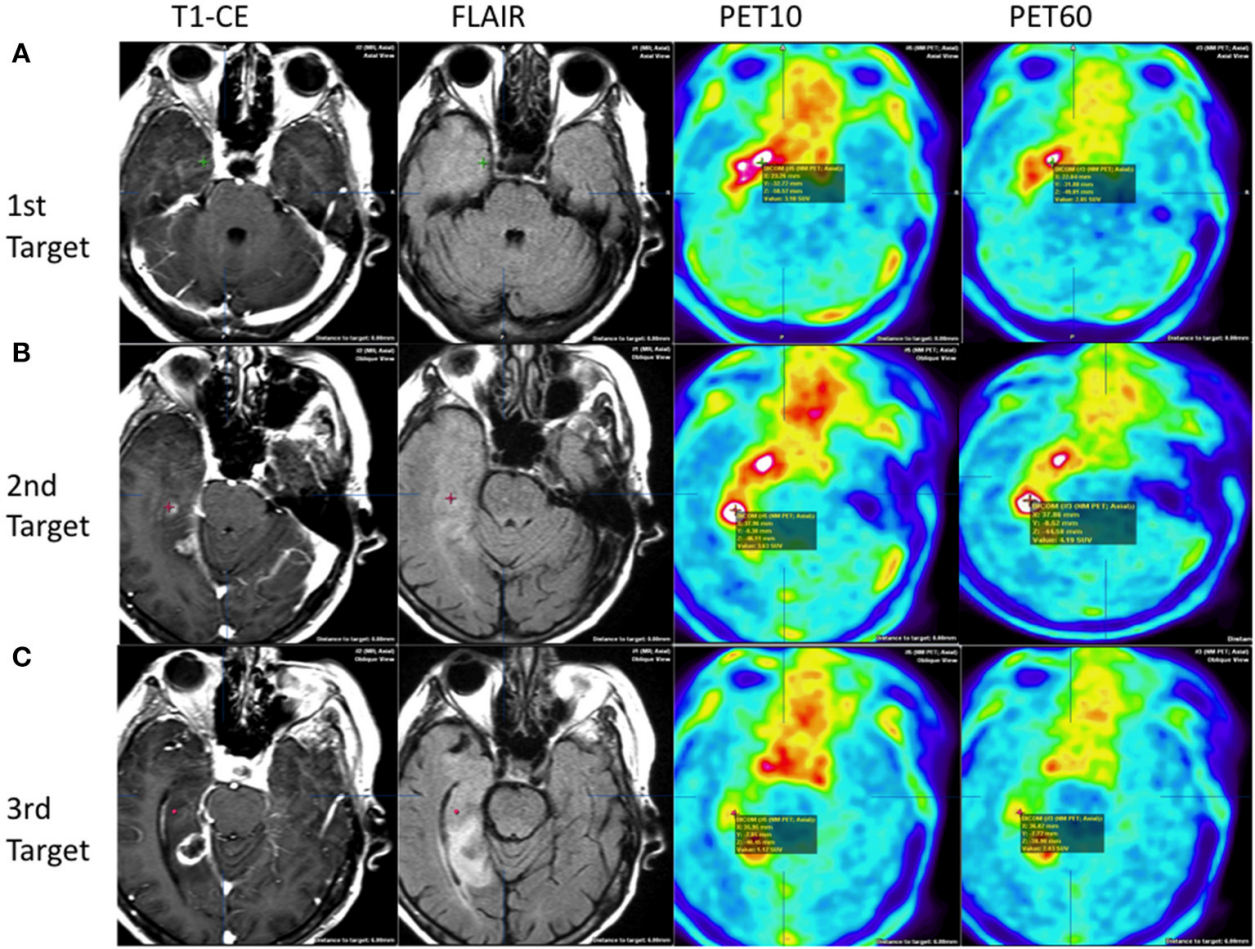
Patient 1: heterogenous tumor biology and uptake behavior. Comparison of three target points in MRI and PET images. Upper row (A) first target based on early PET:WHO IV: without contrast enhancement on T1+CE, normo/hyperintensities on FLAIR, high FET uptake of 3.10 at PET10, and high uptake of 2.85 at PET60. Middle row (B) second target based on MRI, HP: WHO III: with contrast enhancement on T1+CE, hyperintensities on FLAIR, and high FET uptake of 3.63 at PET10 and 4.19 PET60. Lower row (C): third target based on late PET at the tumor periphery HP: WHO III: without contrast enhancement on T1+CE, normointensities on FLAIR, low FET uptake of 1.17 at PET10, and increased up to 2.03 at PET60.
